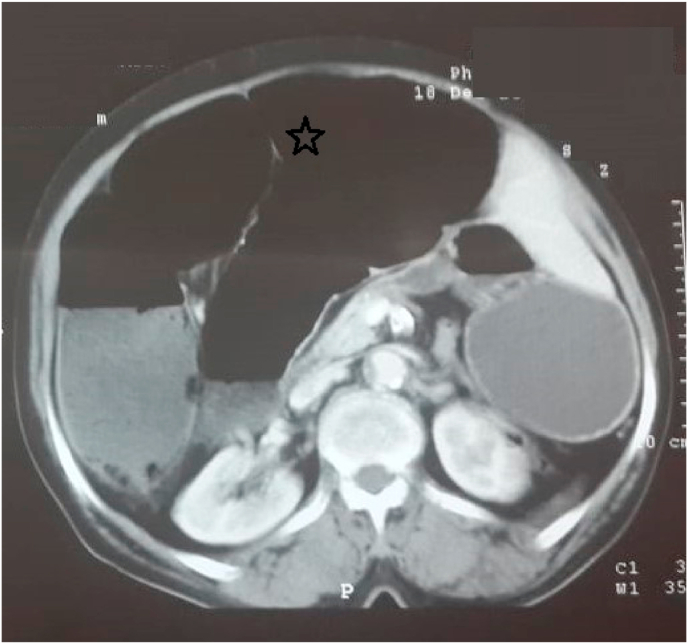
Axial CT image showing a “U-shaped” loop of dilated colon (star).
